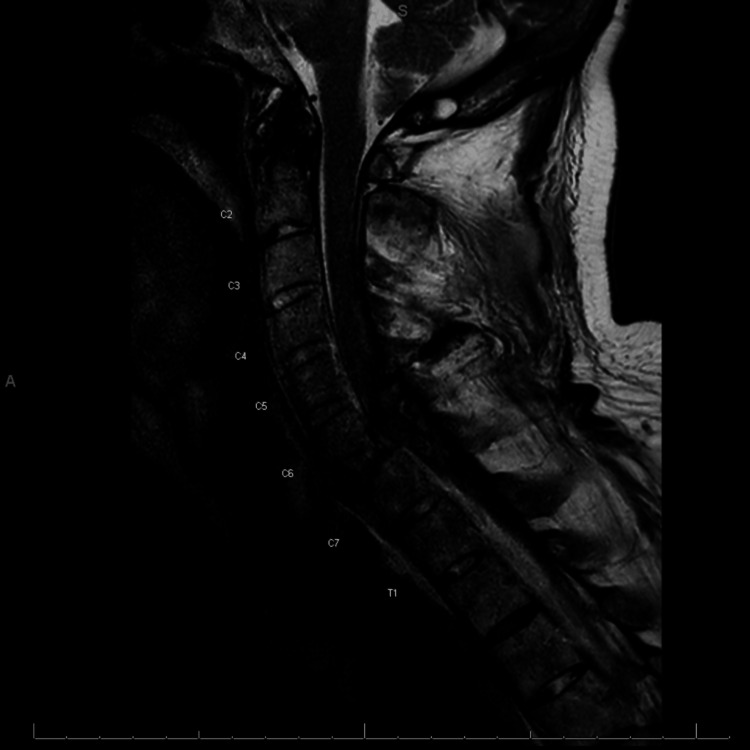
Magnetic resonance imaging (MRI) of the cervical spine showing a chalk-stick fracture from C5 to C7 with a posterior epidural hematoma.
